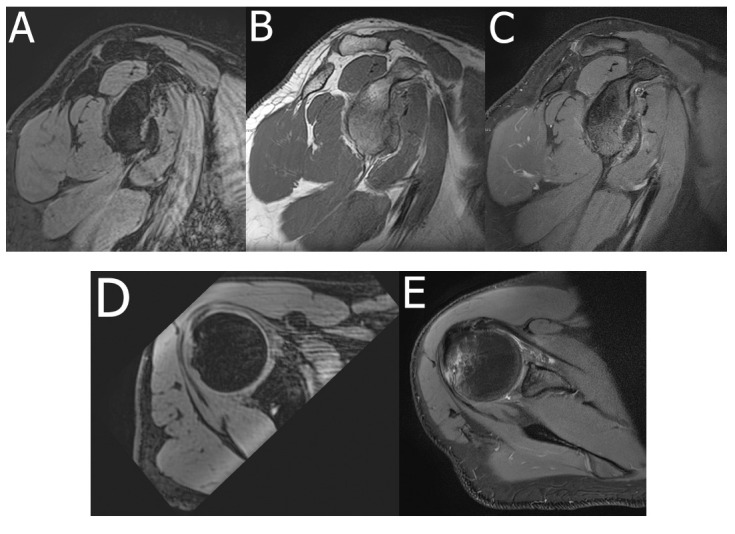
3D MRI for Glenoid track and Hill-Sachs lesion assessment. A 24-year-old patient presented with chronic recurrent shoulder dislocation. Sagittal 3D reconstruction increased spatial resolution for glenoid track assessment (A) compared to conventional 2D T1WI (B) or FS PDWI (C). Hill-Sachs lesion is also better depicted on 3D axial reconstruction (D) compared to FS PDWI (E).
